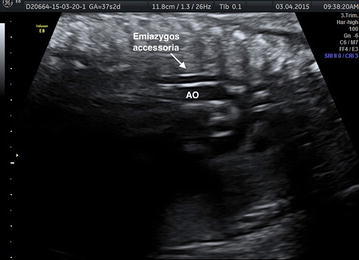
The prenatal ultrasound shows the accessory hemiazygos that drain into the left venous trunk brachial-cephalic
